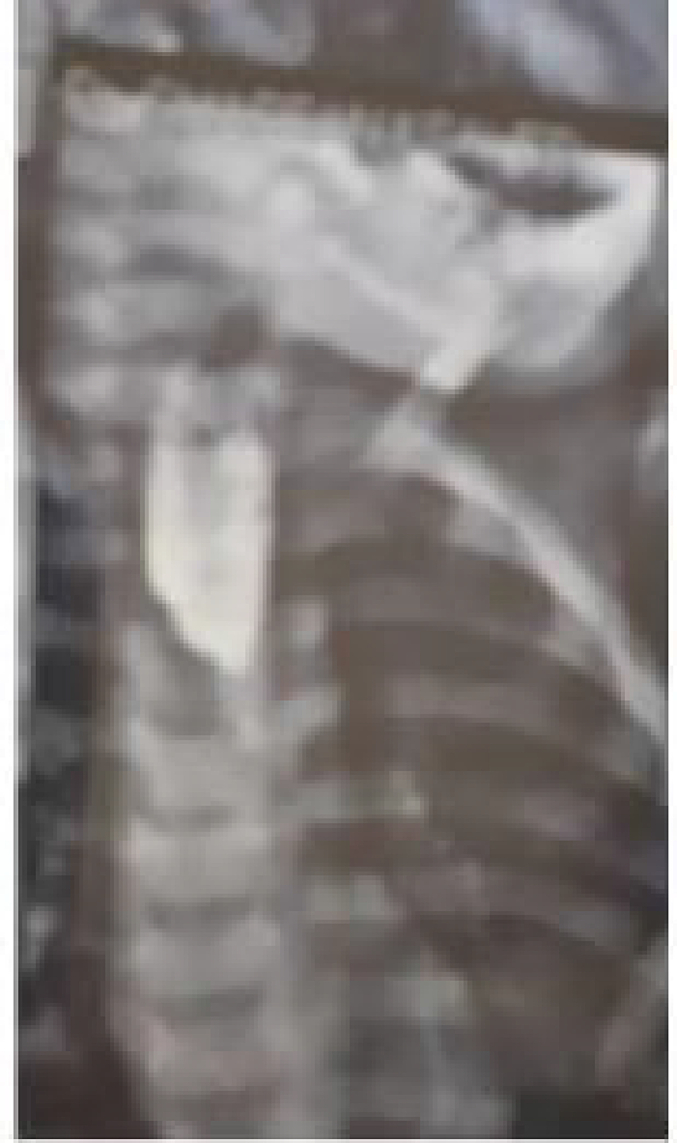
Esophagus and stomach Barium X-Ray in the first visit to hospital shows severe stenosis (the barium gathered in the lower part of the esophagus).
